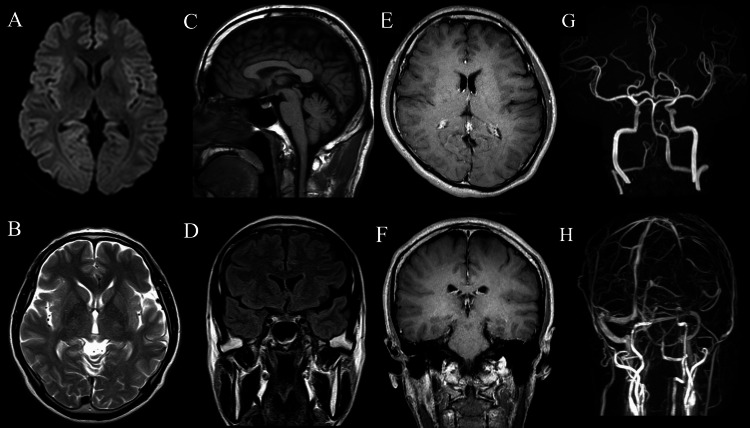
Brain MRI, MRA, and MRV findings Diffusion-weighted imaging shows no acute ischemic lesions (A); Axial T2-weighted imaging reveals no parenchymal abnormalities (B); Sagittal T1-weighted imaging demonstrates normal brain morphology without evidence of structural lesions (C); Coronal fluid-attenuated inversion recovery (FLAIR) imaging shows no abnormal signal intensity (D); Contrast-enhanced axial T1-weighted imaging shows no abnormal enhancement (E); Contrast-enhanced coronal T1-weighted imaging reveals no pathological enhancement (F); Magnetic resonance angiography (MRA) demonstrates no intracranial arterial abnormalities (G); Magnetic resonance venography (MRV) reveals no evidence of cerebral venous sinus thrombosis or venous outflow obstruction (H)
